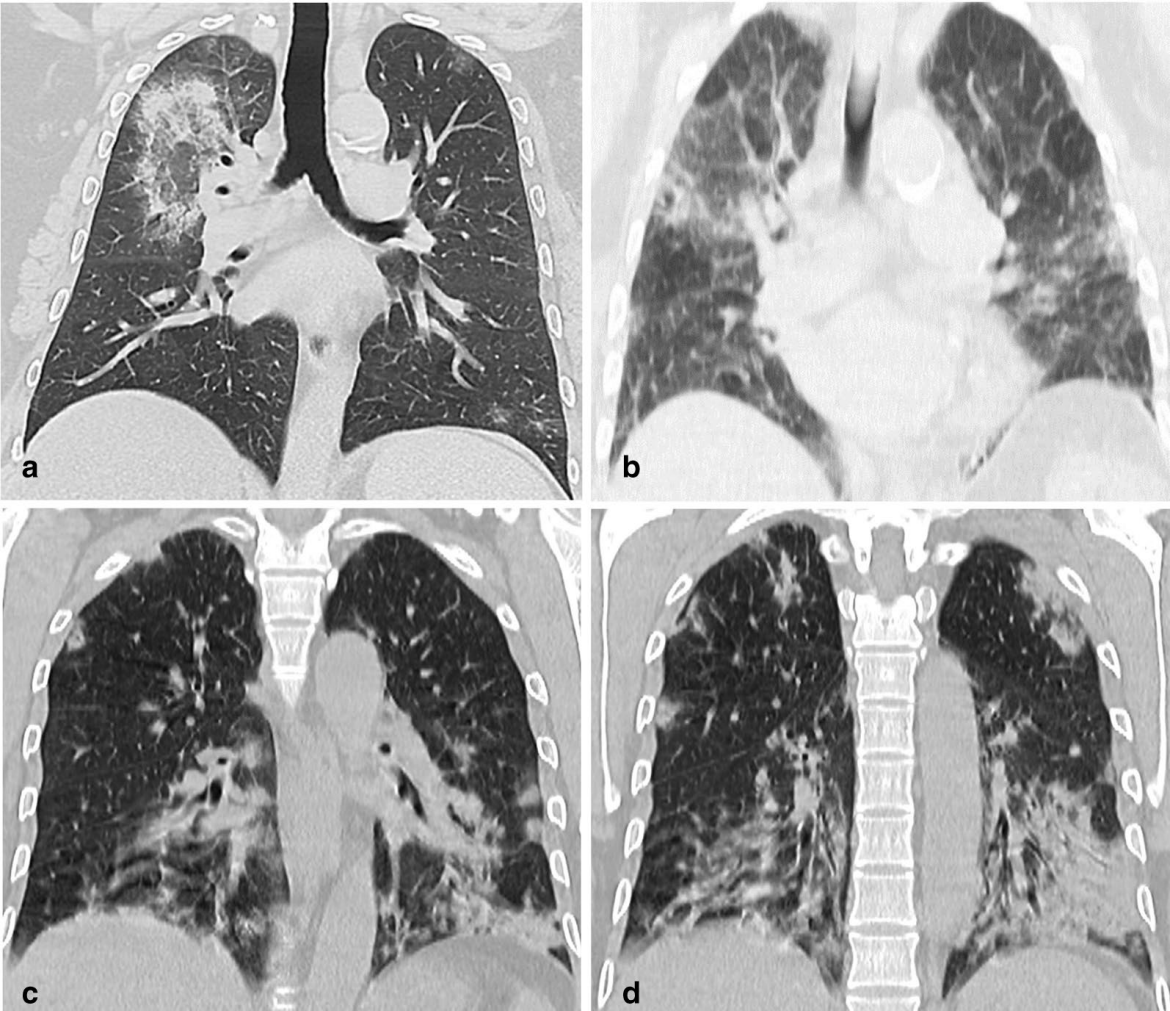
Organizing pneumonia mimicking typical COVID-19. a Cryptogenic organizing pneumonia in a 55-year-old man with history of chest infection not responding to multiple courses of antibiotics, coronal reformatted CT image shows reversed halo sign in the right lung with small areas of GGO in the left lung. Transbronchial biopsy showed findings of COP. b Rheumatoid arthritis induced interstitial pneumonia in a 67-year-old woman, coronal reformatted CT image shows bilateral ground-glass opacities with interlobular and interstitial thickening. c, d Cyclophosphamide-induced OP in a 56-year-old man with Hodgkin disease, coronal reformatted CT images show peripheral multifocal areas of poorly defined focal consolidation, small areas of GGO and bronchial wall thickening
